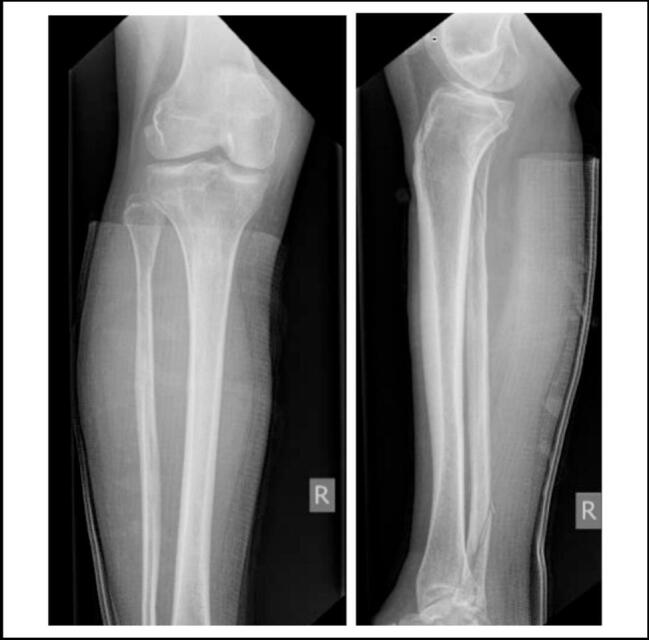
Initial tibial fibular radiographs.
